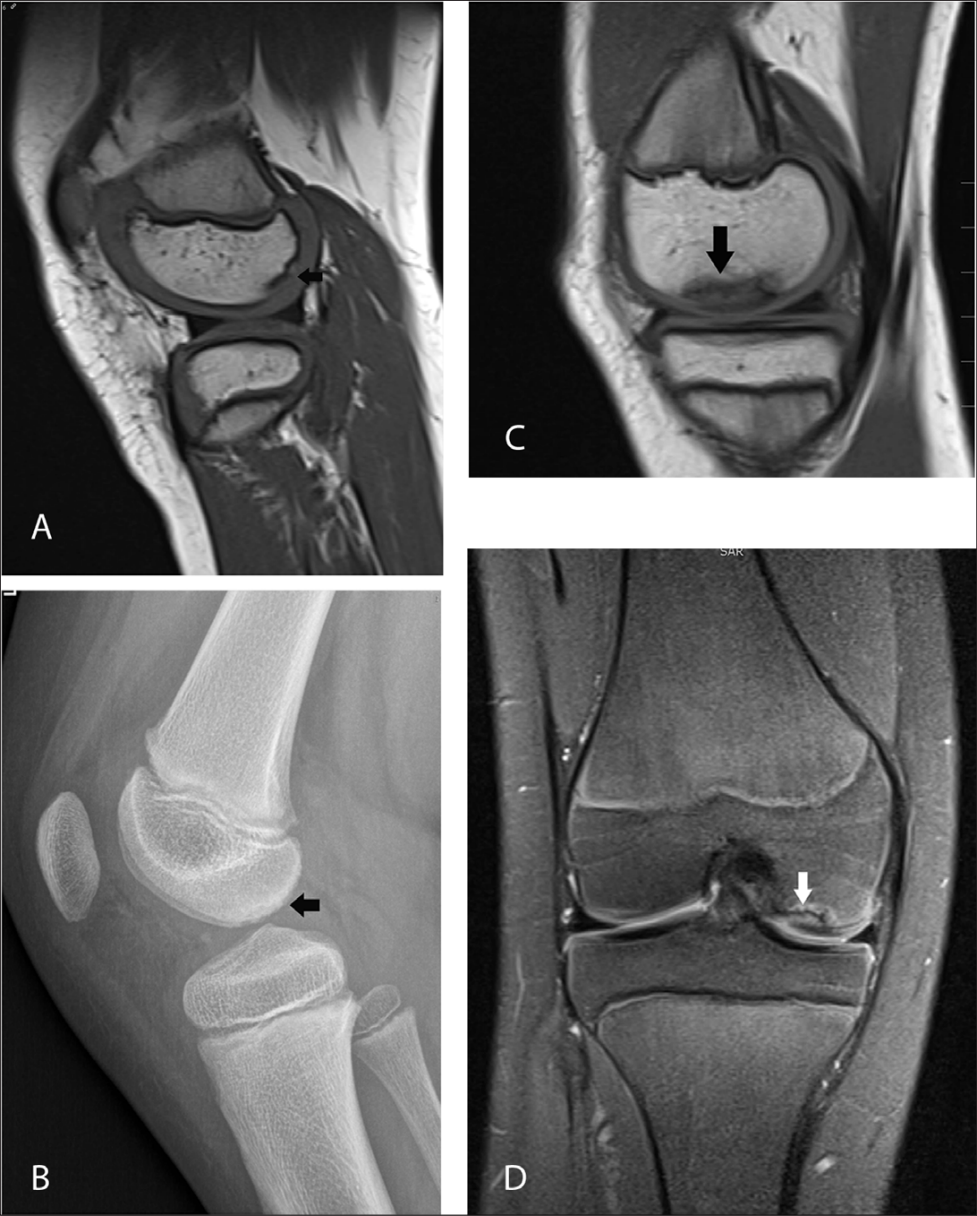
Femoral ossification variant in an 8-year-old boy (A–B) versus osteochondrosis dissecans in a 13-year-old girl (C–D). Sagittal T1-WI (A) and plain films (B) in the first patient show irregular delineation of the subchondral bone plate of the posterior third of the medial femoral condyle with spiculated borders (arrows). Sagittal T1-WI (C) and coronal FS T2-WI (D) in the second patient demonstrate a larger lesion which is located in the medial third (black arrow) of the medial femoral condyle. The lesion is surrounded by bone marrow edema (white arrow). The lateral (inner) border of the lesion extends to the intercondylar roof.
